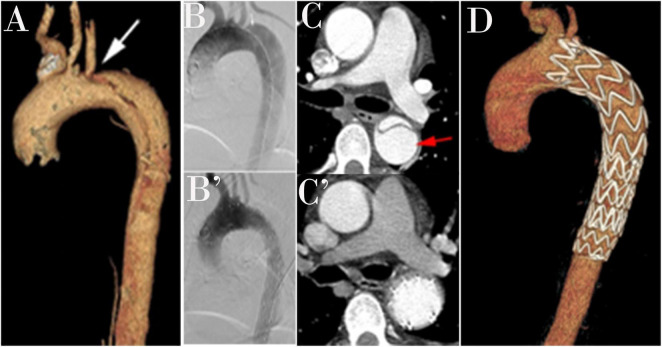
A three-dimensional computed tomography angiography (CTA) is shown in a patient with type B aortic dissection (TBAD; A). An intraoperative angiogram demonstrates TBAD (B). A Post-operative angiogram after thoracic endovascular aortic repair (TEVAR; B’). Preoperative view with a patent false lumen (C). False lumen obliteration after TEVAR (C’). Completion angiogram after successful repair of TBAD shows the coverage of primary entry and no evidence of endoleak (D).
